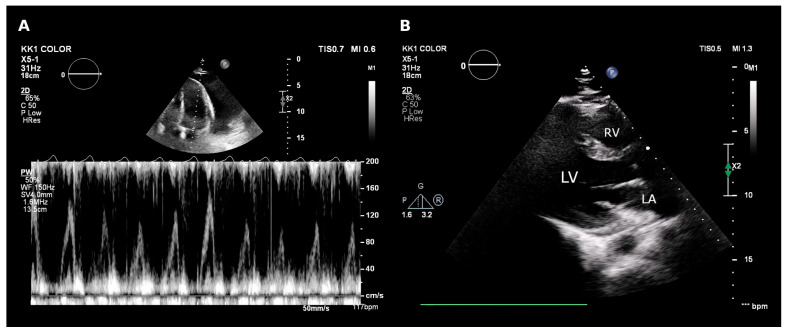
Doppler confirmation of tamponade physiology and postoperative resolution of the effusion. (A) Pulsed-wave Doppler tracing of mitral inflow at presentation (30 + 4 weeks of gestation) showing marked respiratory variation in peak E-wave velocity, consistent with tamponade physiology. (B) Parasternal long-axis view on the first postoperative day, after left anterior mini-thoracotomy with pleuro-pericardial window, showing resolution of the pericardial effusion. LA, left atrium; LV, left ventricle; RV, right ventricle.
